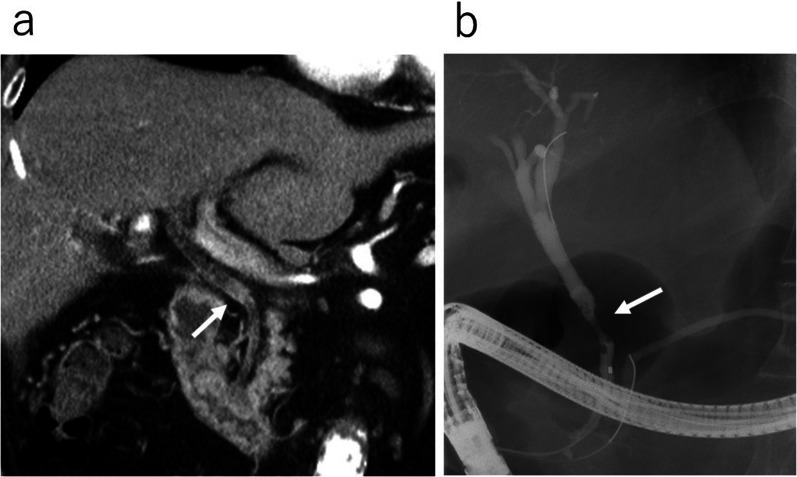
Image findings of distal bile duct cancer. a Contrast-enhanced CT before PD and after percutaneous transhepatic gallbladder drainage (PTGBD) showing a 2-cm-long mass with contrast enhancement in the central bile duct (arrow →). b ERCP before PD showing the narrowing of the distal bile duct (arrow ←) without the visualization of the cystic duct. The cytology of the bile duct brush and bile cytology were both class III
